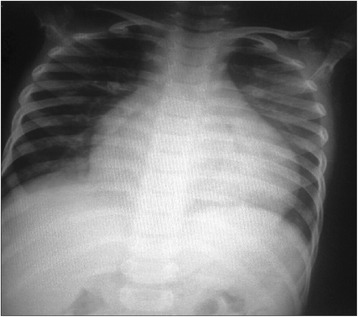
A 6-year-old male child with Carvajal syndrome (variant of Naxos disease): Chest X-ray posteroanterior view showing left-sided cardiomegaly. Original materials from an open access article: [57]
